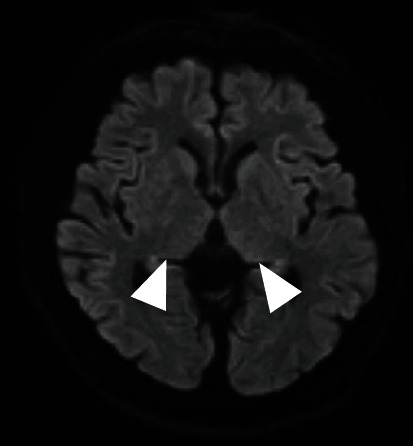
MRI on day 16 revealed no changes in the findings except loss of hyperintensity on DWI (arrow). DWI: diffusion-weighted image; MRI: magnetic resonance imaging.
